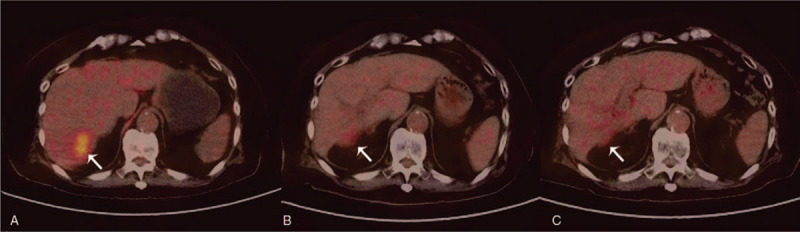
A: PET/CT showed an elevated 18F-FDG uptake (SUVmax 5.1) of the S7 lesion (June 2016). B: Reduction on size and decreased 18F-FDG uptake (SUVmax 2.7) was shown by PET/CT after EBRT 8 months (April 2017). C: Further reduction on size and normalized uptake were shown 1 year after EBRT (October 2017). 18F-FDG = fluorodeoxyglucose. SUV = standard uptake value.
